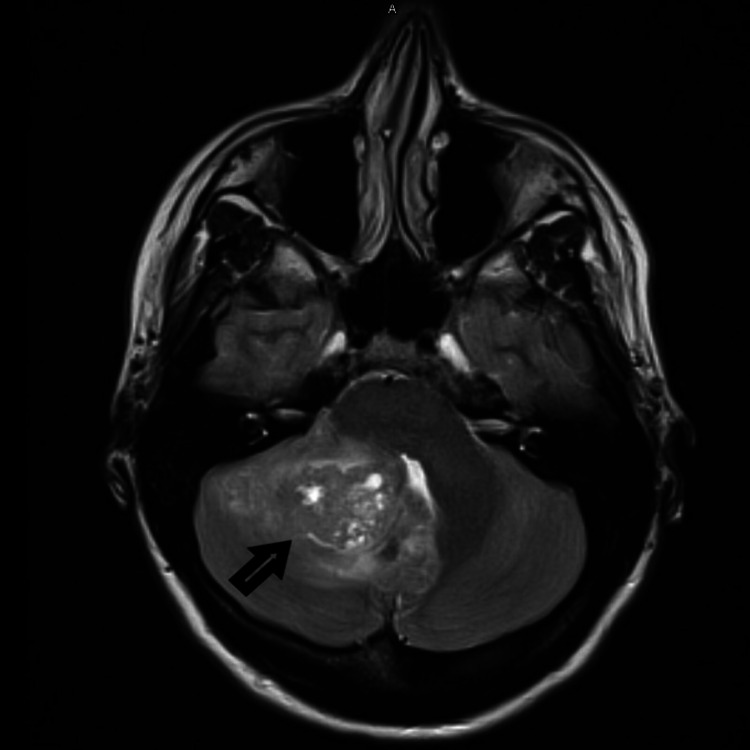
MRI of the brain showing right medial cerebellum mass.
